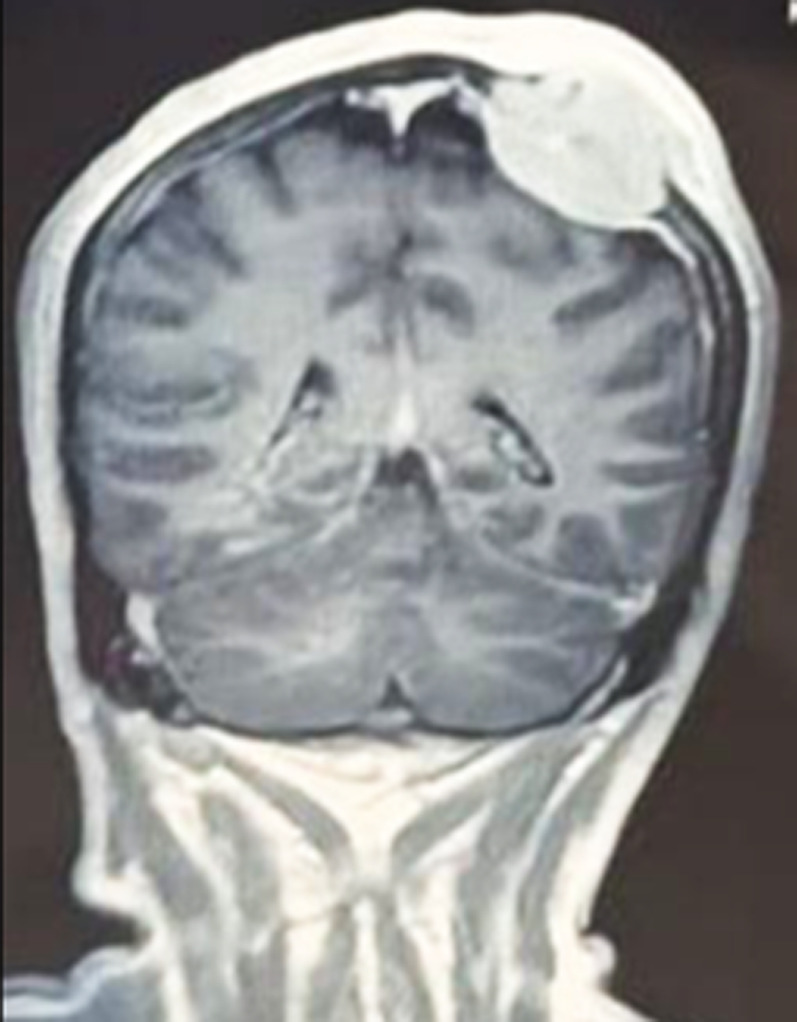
the brain magnetic resonance imaging in coronal section, T1-weighted sequence with gadolinium contrast, reveals a well-circumscribed extra-axial mass located at the right fronto-parietal convexity; the lesion demonstrates marked homogeneous enhancement and is in continuity with the dura mater; it exerts a mass effect on the adjacent cerebral parenchyma, causing mild displacement of the midline structures, with no evidence of parenchymal invasion visible on this section
